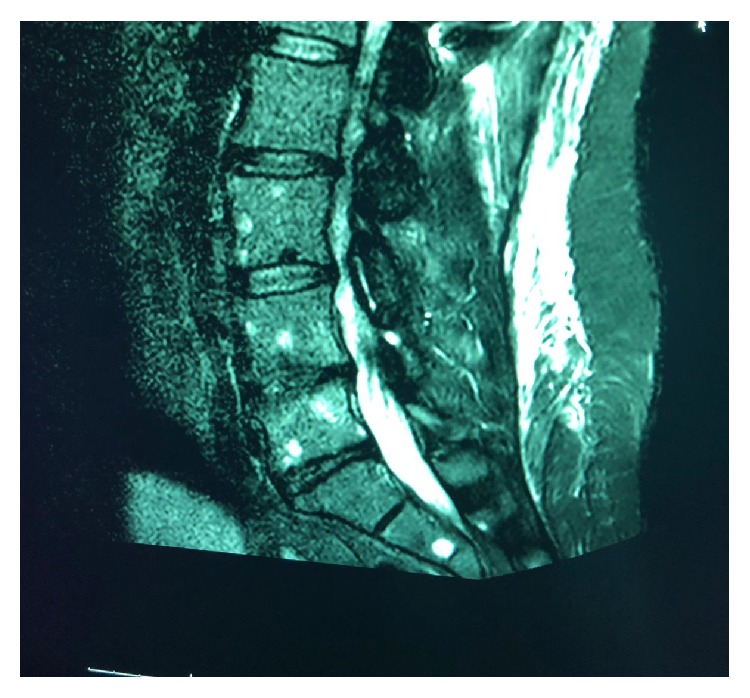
MRI shows multiple, variable-sized, well-defined round lesions.
